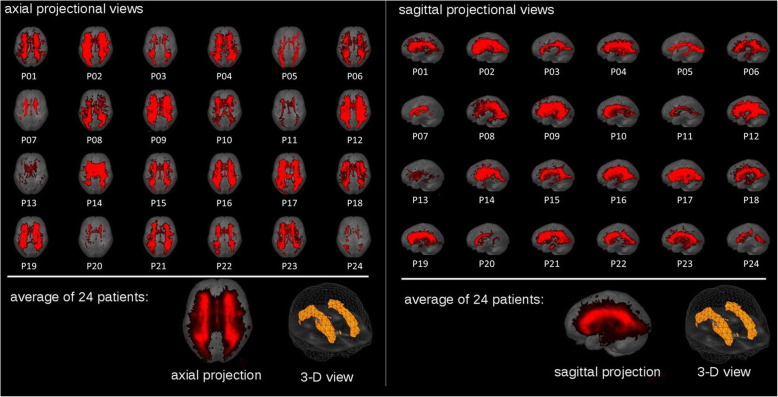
White matter hyperintensities (WMH) for 24 patients detected from FLAIR-MRI-recordings in axial (left) and sagittal (right) projectional views. For comparability, individual results were normalized to the Montreal Neurological Institute (MNI) coordinate frame and displayed on the same morphological background (segmented averaged brain)
